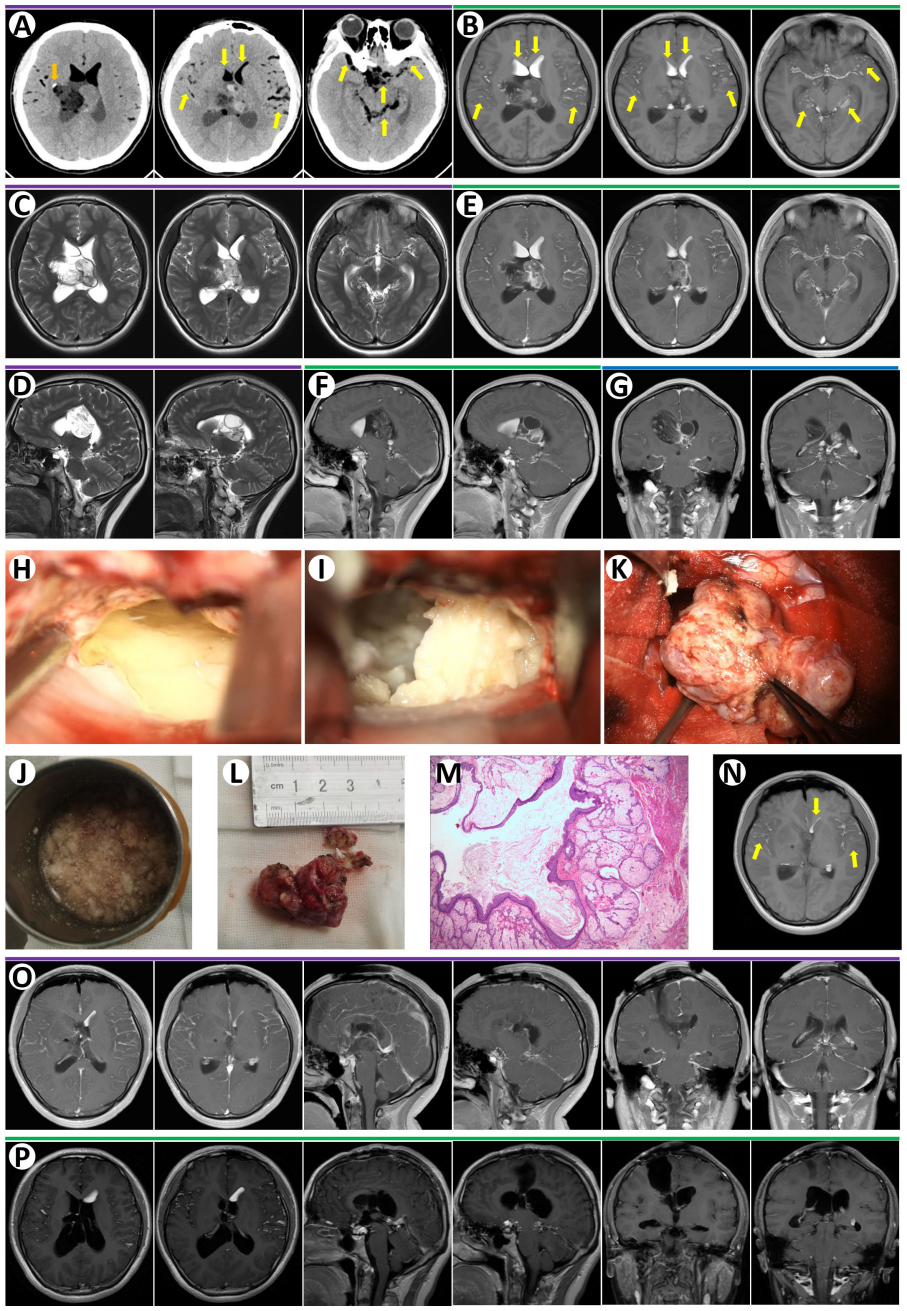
(A) Preoperative non-contrast-enhanced CT revealed a right lateral ventricle lesion with calcium in right rim (orange arrow). There were many low-density signals in bi-lateral ventricles and subarachnoid space likely oil or air (yellow arrows). (B) Axial T1-weighted MRI images demonstrated multiple hyperintense signals, oily globules, in ventricles and subarachnoid space (yellow arrows). Axial T2-weighted MRI images showed a lesion with mixed signal in the right lateral ventricle and septum pellucidum (C, axial; D, sagittal). T1-enhanced MRI images revealed heterogeneous enhancement of the lateral ventricle lesion and strong enhancement of septum pellucidum lesion (E, axial; F, sagittal; G, coronal). (H) The oily substances floating in CSF were observed during microsurgery. (I) The lesion containing cottage-cheese-like material was removed during microsurgery. (K) Gross total resection of the lesion during microsurgery. The oily substances in the cup (J) and the resected lesion (L) were demonstrated. (M) Paraffin section of this lesion confirmed mature teratoma. (N) Axial T1-weighted MRI image at day 1 after surgery revealed oily globules in ventricles and subarachnoid space (yellow arrows). (O) T1-enhanced MRI images at day 1 after surgery confirmed the gross total resection of the tumor. (P) T1-enhanced MRI images in 15 months after discharge showed no recurrence.
